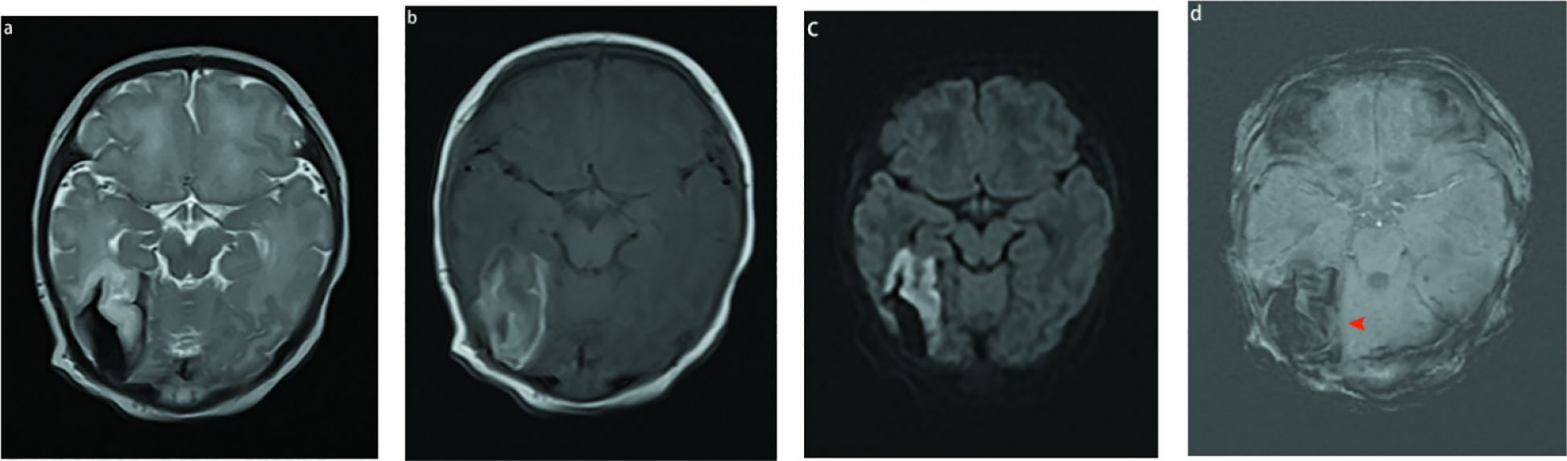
MR image of a full-term neonates with pattern C. The subpial hemorrhage shows hypointense on T2WI and hyperintense on T1WI. Hemorrhage is seen in the underlying cerebral cortex and white matter, resulting in a hyperintense on T1WI and T2WI (a-b). There was no restricted diffusion in the subpial bleed and hyperintense on underlying cerebral cortex and white matter (c). SWI shows magnetic susceptibility artifact blurs, making it diffcult to separate the subpial hemorrhage from underlying the cortical ribbon ( d, arrowhead). This combination of a dark subpial hemorrhage collection and the bright underlying cerebral cortex and white matter forms a yin-yang sign (a and c).
